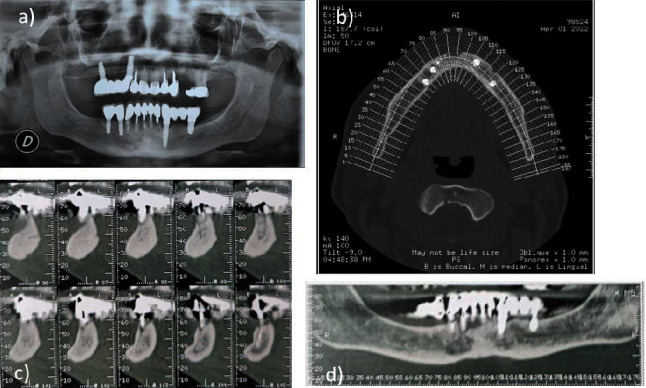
Radiographic examinations performed in March 2022: (a) the OPT showed the presence of several dental treatments and an area of radiolucency below the lower incisors; CBCT revealed the presence of bone sequestration area; (b) axial cuts of the CBCT scan; (c) sagittal cuts of the CBCT; (d) coronal cuts of the CBCT.
